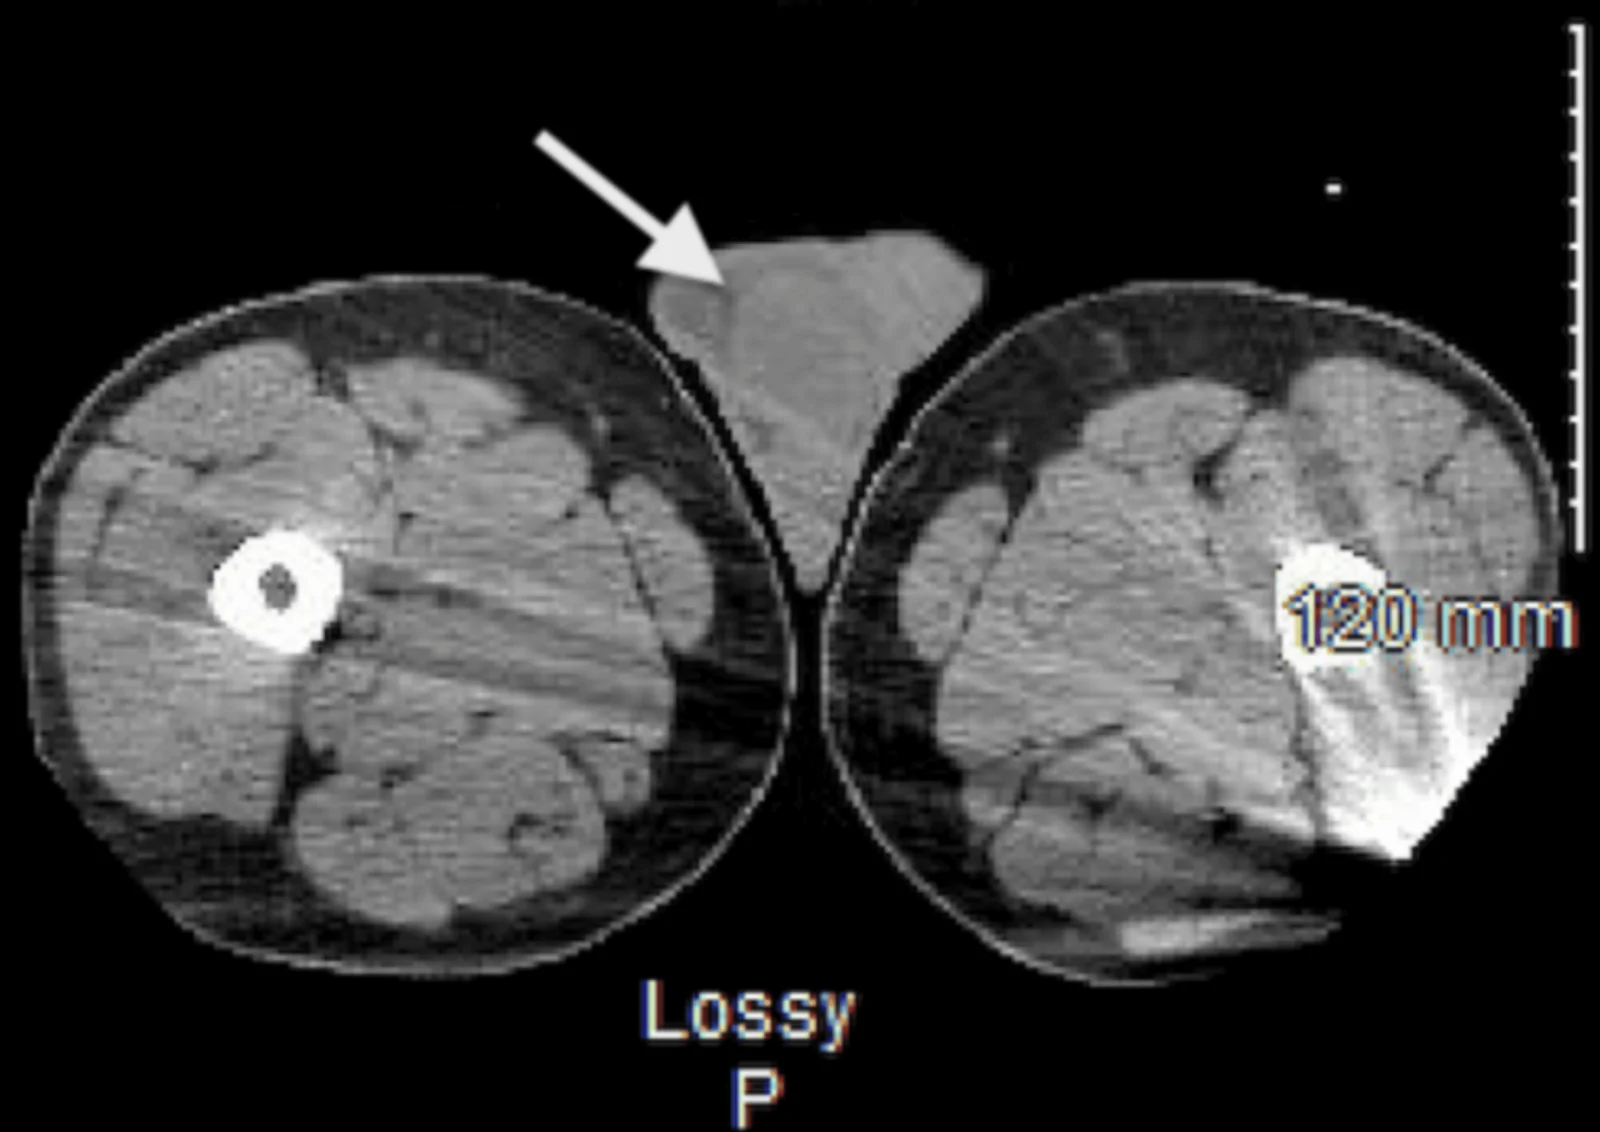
Inferior testicular displacement on preoperative imaging. CT demonstrates inferior displacement of the right testis within the right hemiscrotum (white arrow). Suprapubic testicular displacement was not present on this image and was identified only during surgery approximately 48 h later.
